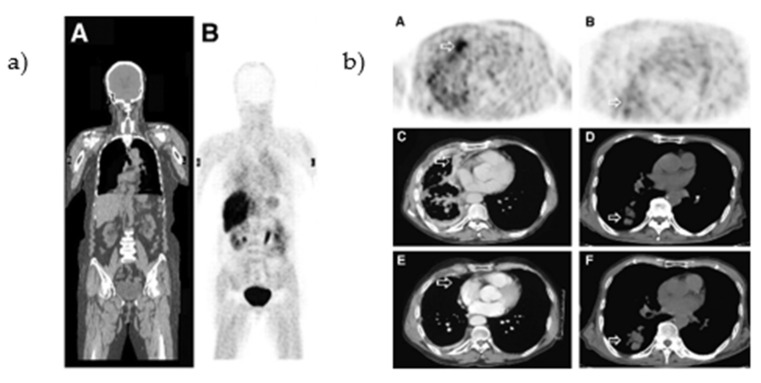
(a) [*O-methyl*-^11^C]PD153035 whole-body distribution 30–40 min after injection in a healthy volunteer. Adapted from [44], with permission from SNMMI. (b) Baseline [*O-methyl*-^11^C]PD153035 PET in (A) an adenocarcinoma patient and (B) a squamous cell carcinoma patient, with corresponding CT before treatment (C,D) and 6 weeks after treatment (E,F). Adapted from [46], with permission from SNMMI.
